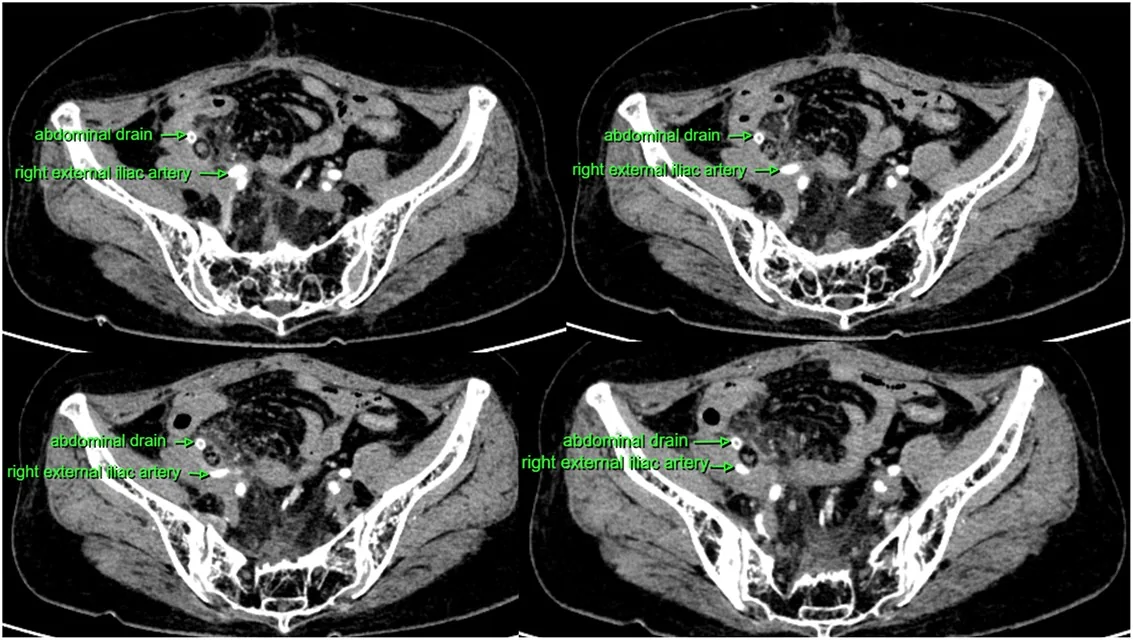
Contrast-enhanced CT (arterial phase) at the time of active bleeding, one month after open surgical removal of the foreign body. The arrows mark the right external iliac artery and the pelvic drainage tube, respectively. No definite pseudoaneurysm or active extravasation is identified.
